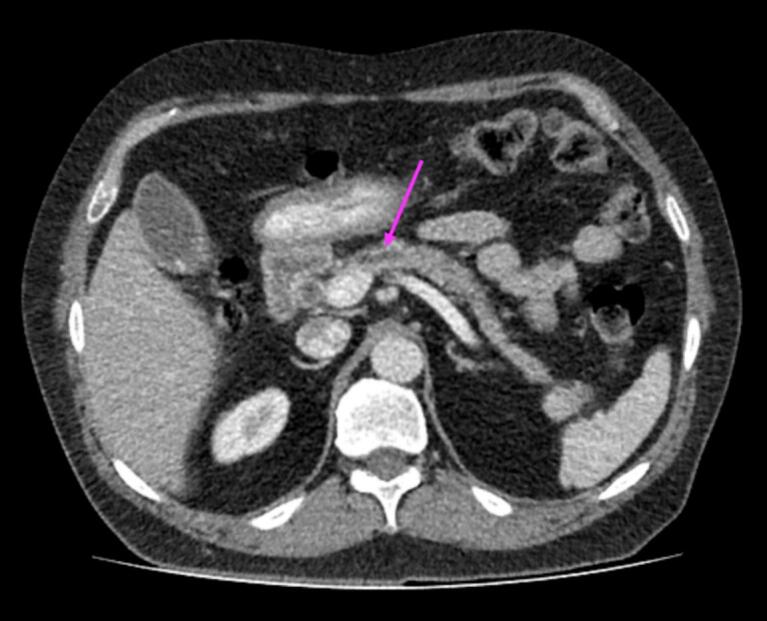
Irregular-shaped hypoechoic lesion at the head of the pancreas in contrast-enhanced Abdominal CT scan, Portal phase.
